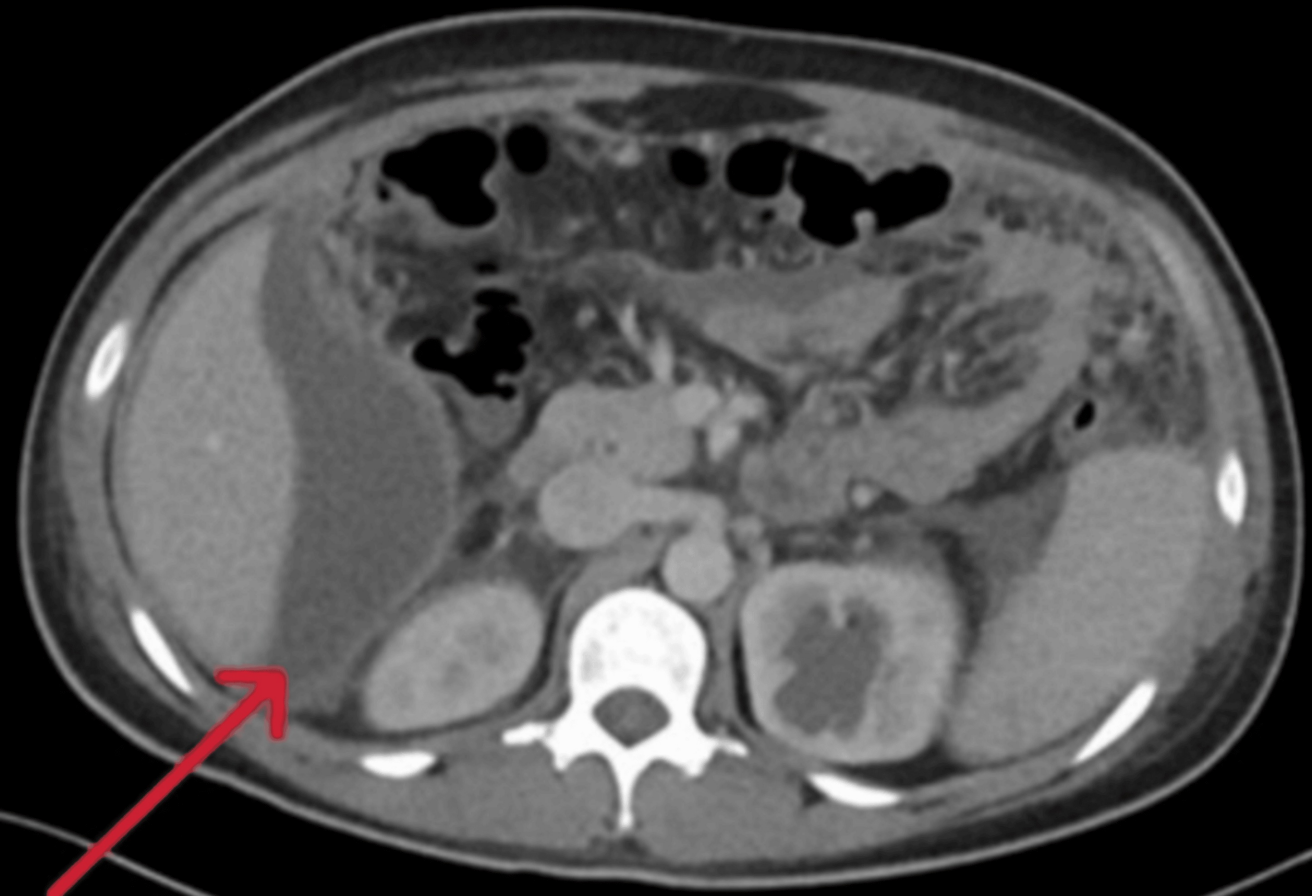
CT scan/axial view revealed subhepatic collection (red arrow)
